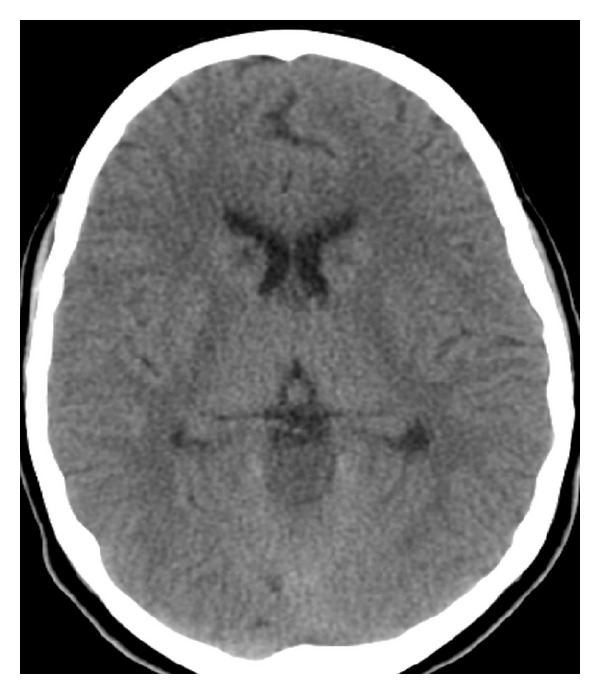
Axial images of Computed Tomography (CT) of brain showing bilateral hypodensities of the caudate nuclei.
